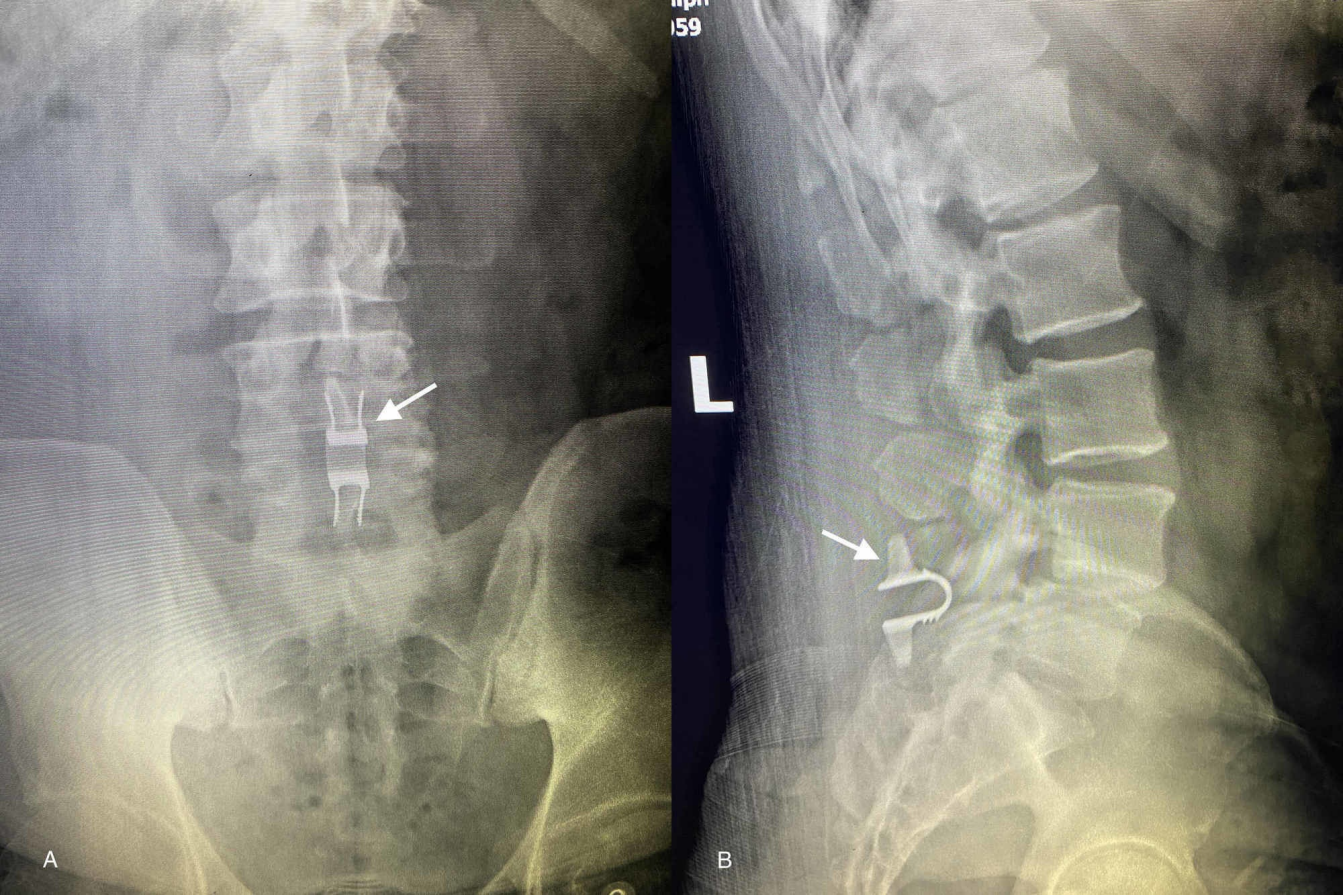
Two week postoperative Coflex® ILS implant radiographs Anteroposterior (A) and lateral (B) radiographs obtained two weeks postoperatively showing Coflex ILS implant (white arrows) in appropriate position at the L4-L5 level. ILS: Interlaminar stabilization
